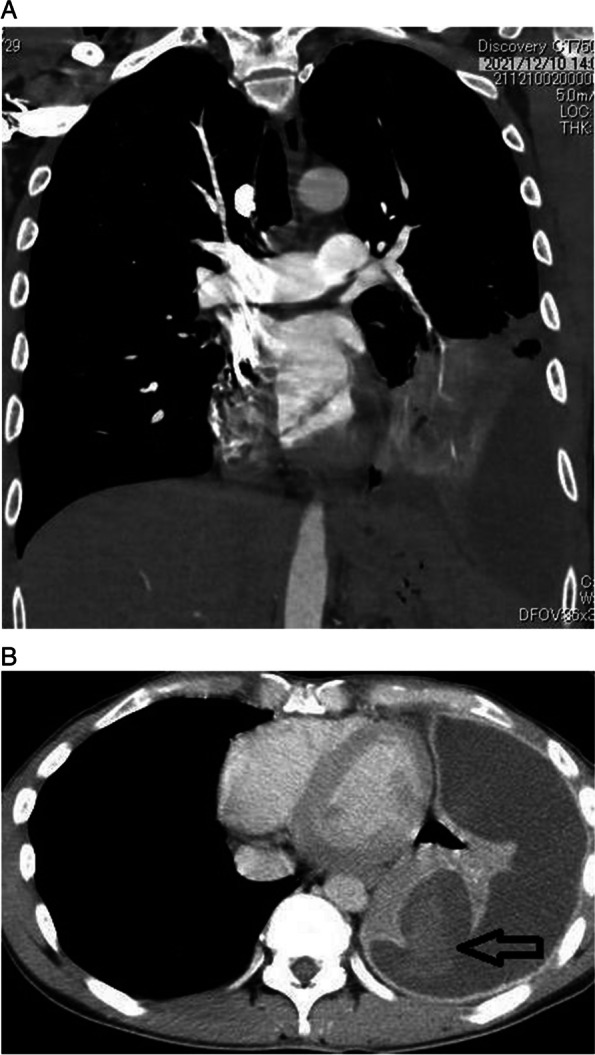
a Chest CT showing a left empyema due to ruptured lung abscess in the left lower lobe. b A pale high-concentration hematoma is suspected in the perforation (arrow), but no obvious extravasation is observed
